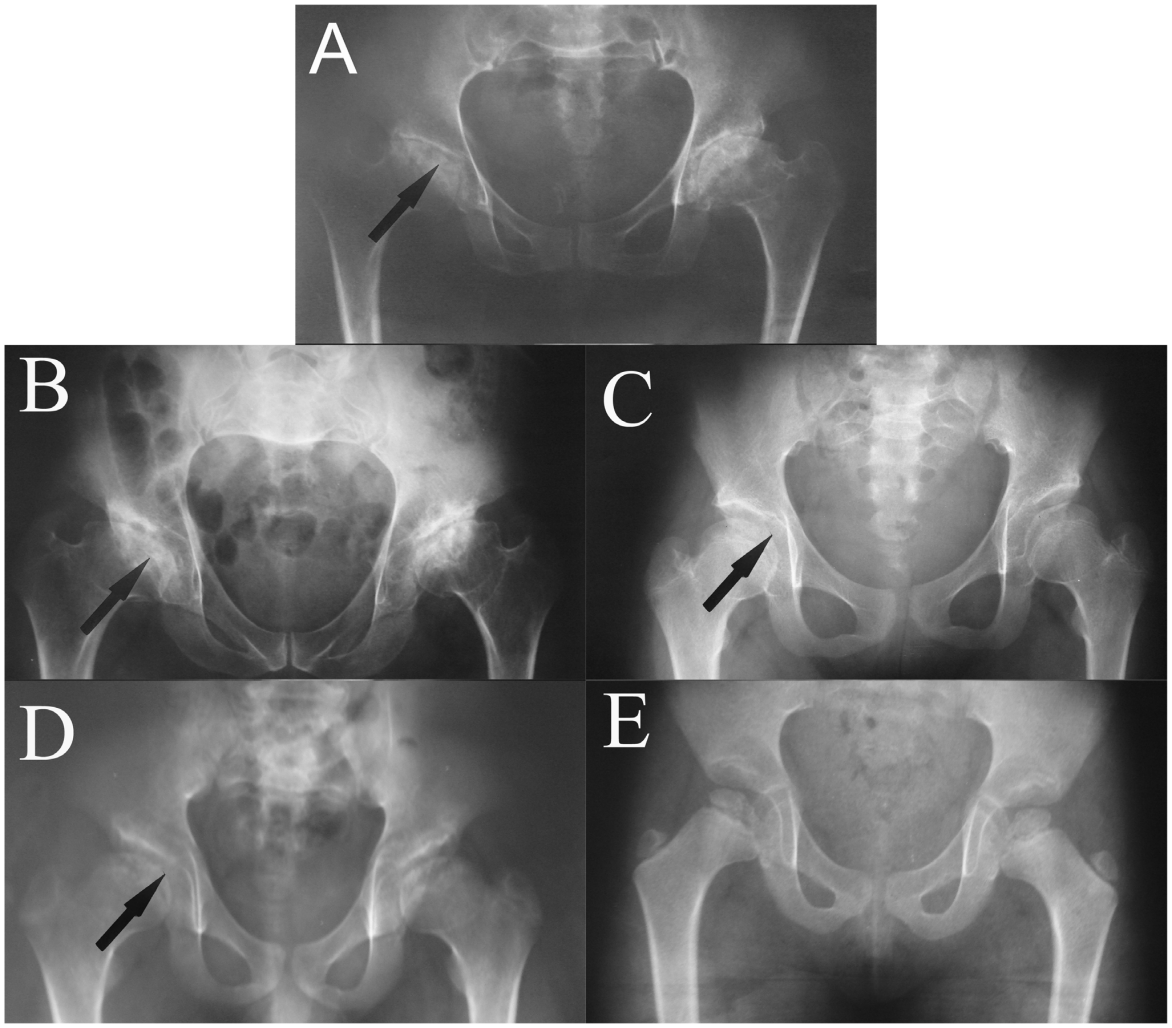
Radiograph of the family members. Radiographs of the pelvis of the family members III-6 (A) and III-7 (B), diagnosed with LCPD, reveals abnormalities of the capital femoral epiphyses, flattening of the acetabular roof with cystic degeneration, coxa plana (coxa vara and elevation of the greater trochanter of the femur) (arrow). The radiographs of the hip in family member IV-7 (C) and IV-8 (D) shows partial collapse of the femoral head, without coxa plana (arrow); the patient was diagnosed with ANFH. The radiograph of the family member IV-9 (E) did not exist typical manifestations. In addition, The X-ray of the family members III-6, III-7, IV-7, IV-8, IV-9 were made at the age of 41, 38, 15, 15 and 7 years old respectively.
